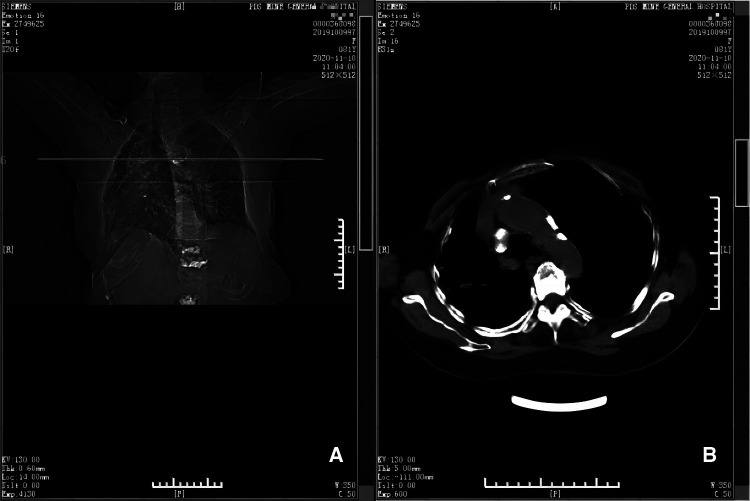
CT scan taken at 12th month postoperatively (panels A and B). Panel A depicts the position (horizontal line) of the CT scan shown in B, at the level of the 5th thoracic vertebra. Panel B shows there is no hematoma near the 5th thoracic vertebra and adjacent ribs.
